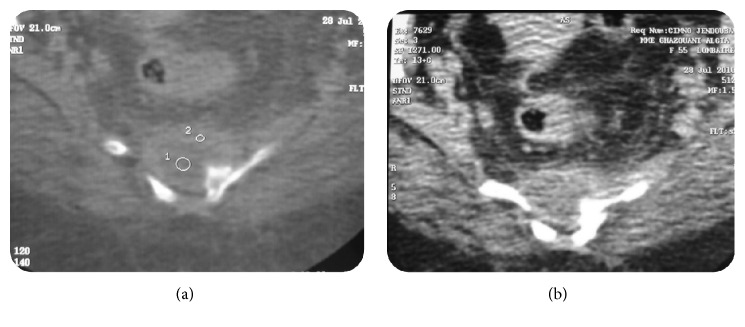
Contrast-enhanced computed tomography (CT) scans of the patient revealing presacrococcygeal lytic process.
